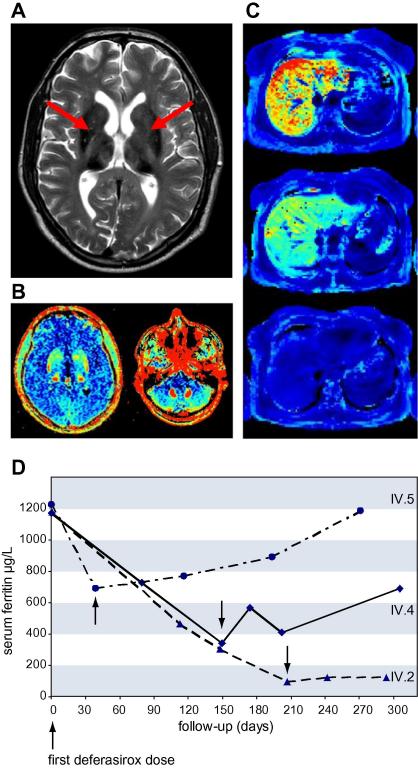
Iron accumulation and results from deferasirox treatment. (A) T2 brain MRI of patient IV.4 shows a marked hypodensity in the area of the basal ganglia indicating severe iron accumulation (arrows). (B) T2∗ maps of the brain confirm the significant iron accumulation in the basal ganglia and show iron loading in the dentate nucleus (red colour indicates areas with a high and dark blue colour those with a low tissue iron concentration). (C) Abdominal T2∗ maps of patient IV.4 show liver iron overload of approximately 250 μmol Fe/g liver tissue before deferasirox treatment. After 2 months of treatment a reduction in liver iron concentration was found and no visible liver iron remained after 5 months of treatment (25 μmol Fe/g liver tissue). (D) Serum ferritin concentrations rapidly decreased during deferasirox treatment. Arrows indicate the first visit after cessation of iron chelation therapy.
